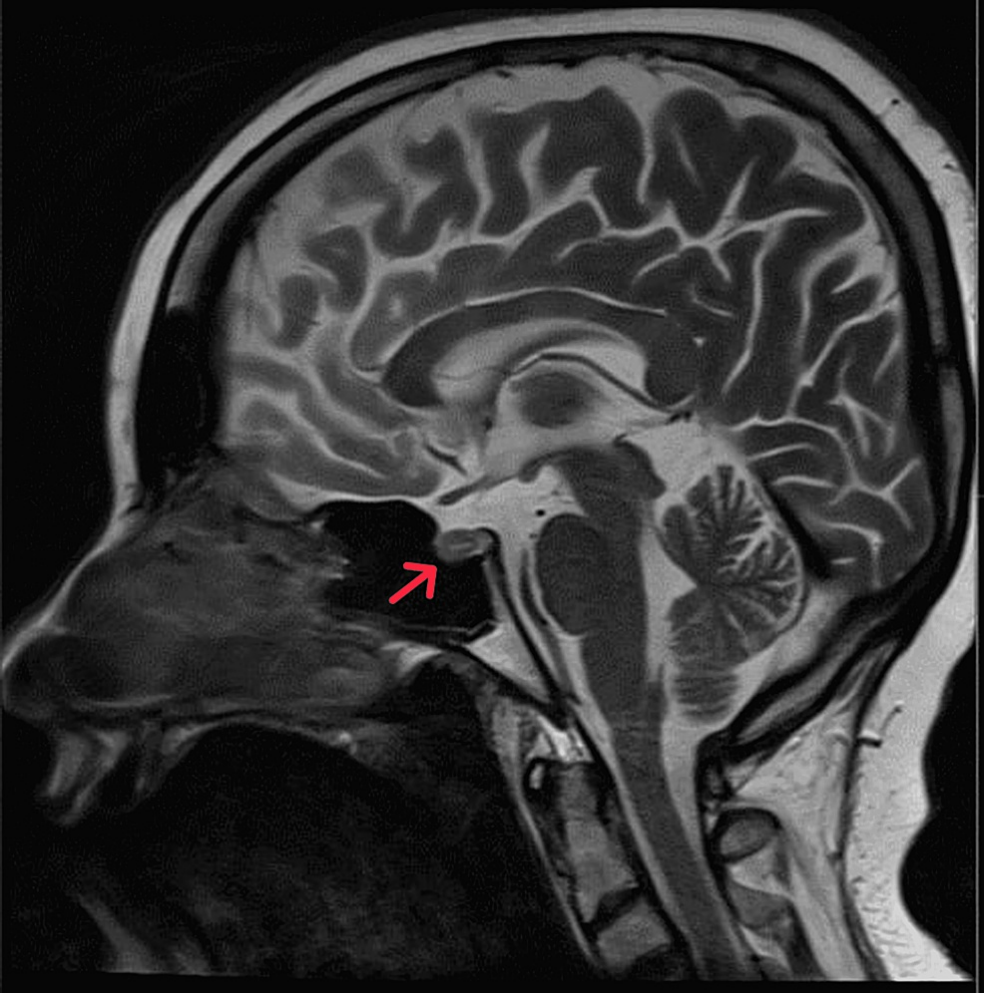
Brain MRI sagittal view showing focal anterior pituitary hypoenhancing lesion at the midline and eccentric to the right
